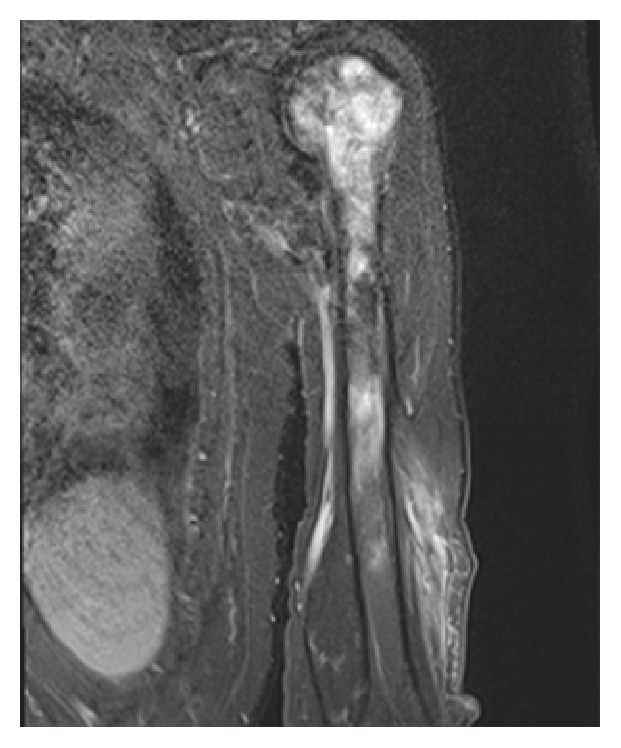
MRI of the left shoulder demonstrates a soft tissue lesion adjacent to the proximal humerus and potential bony lesions.
